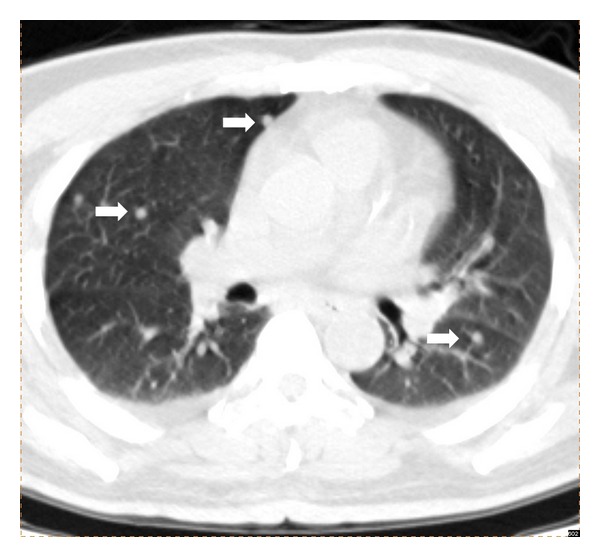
CT image from a 48-year-old male patient diagnosed with hepatocellular carcinoma. The followup unenhanced CT of ^18^F-fluoride PET/CT, in the lung window, revealed numerous tiny pulmonary nodules in the bilateral lungs (arrows). The largest nodule was 0.6 cm in size and none of these nodules were visualized by concurrent chest X-ray.
